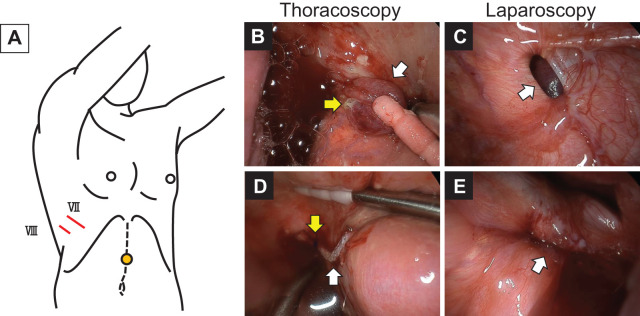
Surgical setup and intraoperative thoracoscopic and laparoscopic findings. (A) The chest tube placed at the 8th intercostal space along the midaxillary line was removed, and the incision was extended to serve as the initial thoracoscopic camera port. Under thoracoscopic guidance, a 4-cm utility incision was made along the anterior axillary line at the 7th intercostal space, allowing a 2-port approach. Given the history of prior laparotomy and anticipated extensive intra-abdominal adhesions, preoperative abdominal ultrasound was performed to assess adhesion sites. A camera port was placed at a site where mild ascites provided sufficient separation between the abdominal wall and the intra-abdominal organs (yellow circle). (B) Thoracoscopy revealed a 1-cm diaphragmatic hernia sac (white arrow). A pneumoperitoneum test using CO_2_ insufflation demonstrated air leakage from a pinhole defect within the hernia sac (yellow arrow), confirming the presence of a pleuroperitoneal communication. (C) Laparoscopy identified a 1-cm diaphragmatic defect in the central tendinous portion of the right diaphragm, without evidence of organ herniation (white arrow). (D) The hernia sac was resected using an endoscopic stapler (white arrow). Persistent air leakage on repeated pneumoperitoneum testing necessitated additional sutured closure (yellow arrow). (E) Laparoscopic assessment confirmed the absence of herniated abdominal organs (white arrow).
